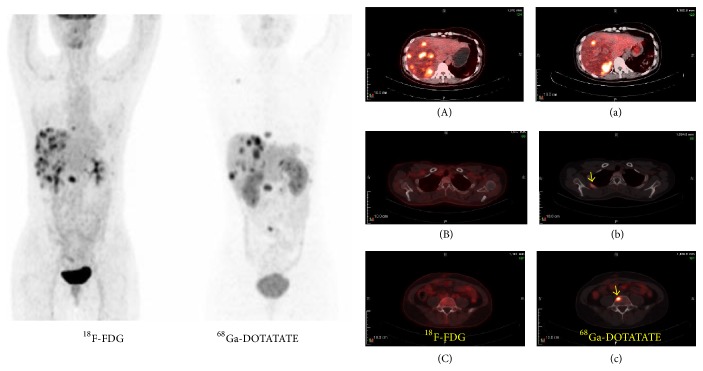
A 37-year-old women with pancreatic NEC G3 (Ki-67 = 80%) and lymph node, liver, and bone metastases, from whom the primary lesion has been resected.^ 18^F-FDG PET/CT showed more liver lesions, while ^68^Ga-DOTATATE detected more bone lesions. ((A) and (a)) Liver lesions showed heterogeneity in SSTR expression. ((B) and (b) and (C) and (c)) ^18^F-FDG PET/CT failed to show bone metastases in rib and lumbar vertebra.
